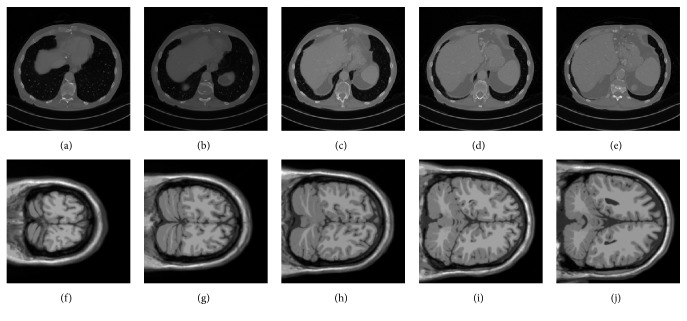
Image samples from our experiments: (a)–(e) CT images, (f)–(i) MR images.
